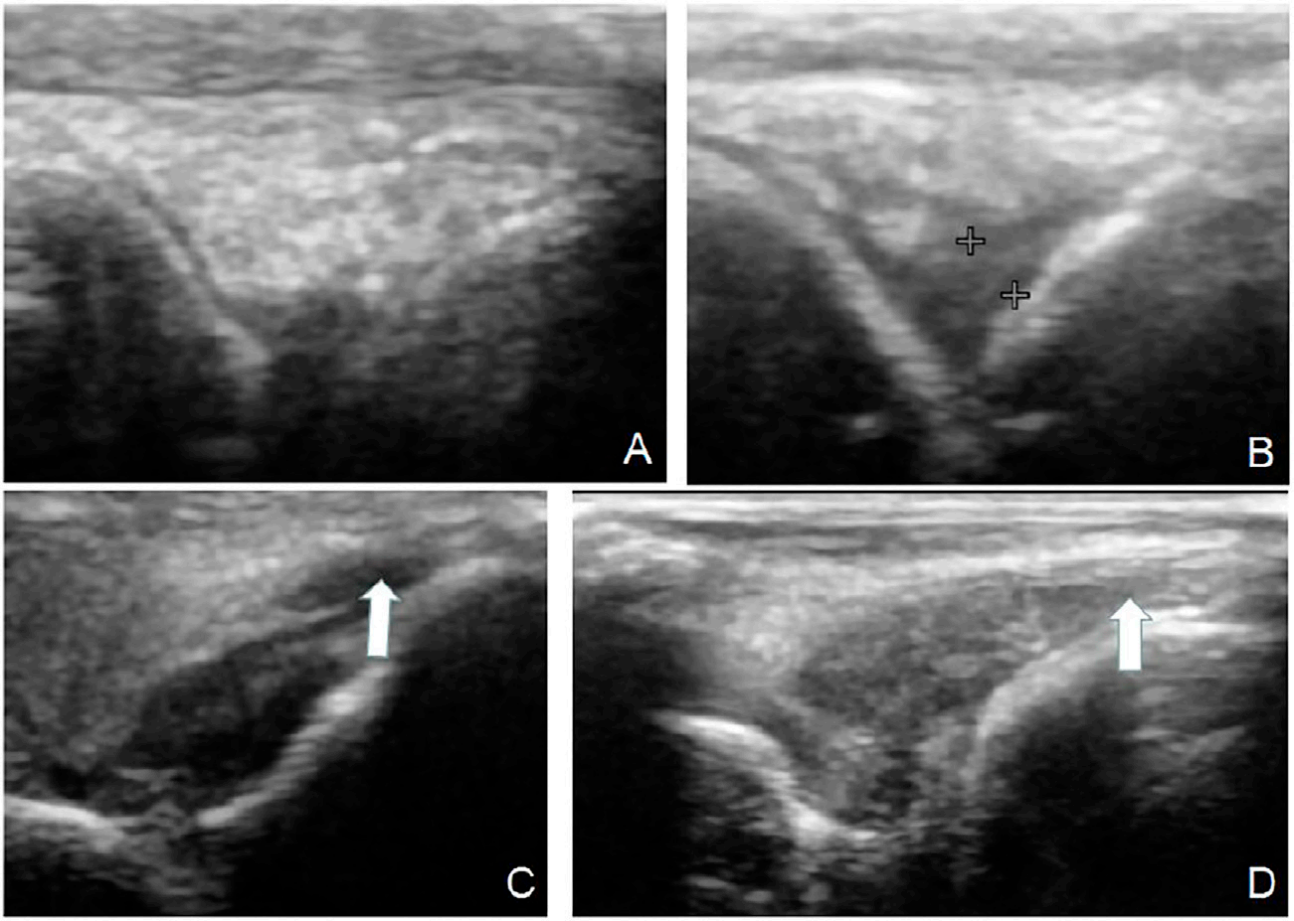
Ultrasonic evaluation of synovitis. Score 0: no obvious thickening of synovium. (TP-SLN group, (A)); score 1: mild synovitis can be seen (+ +), but it does not exceed the line of the highest point on the bone surface (TP group, (B)). Score 2: the line is higher than the highest point of bone surface, but not higher than the backbone (DMSO group, (C)). Score 3: over the line of the highest point of the bone surface and extending over one side of the shaft (control group, (D)).
